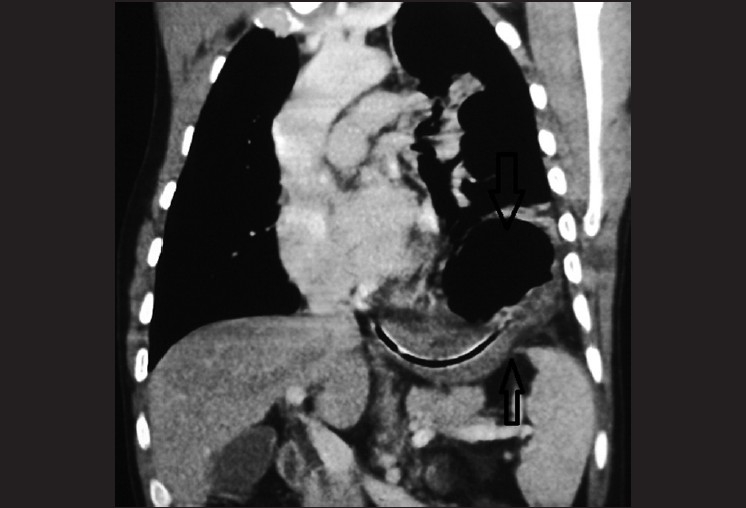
Coronal reformat computed tomographic image showing a defect in the left hemidiaphragm with the stomach containing the nasogastric tube within the defect (upward arrow), with the stomach being dilated superior to it inside the left hemithorax (downward arrow)
